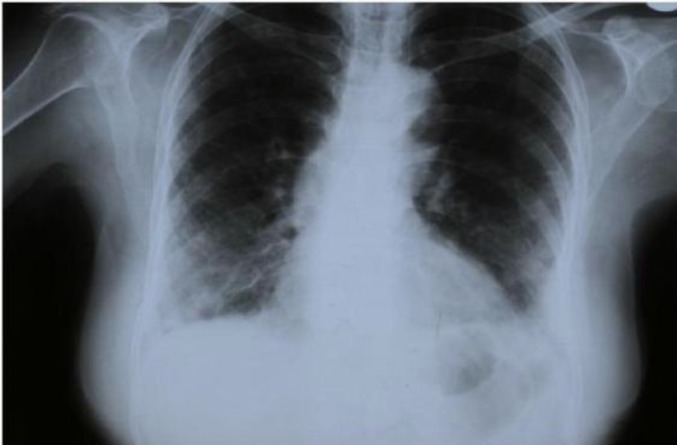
Chest x-ray (CxR) Showing not any pathologic sign
